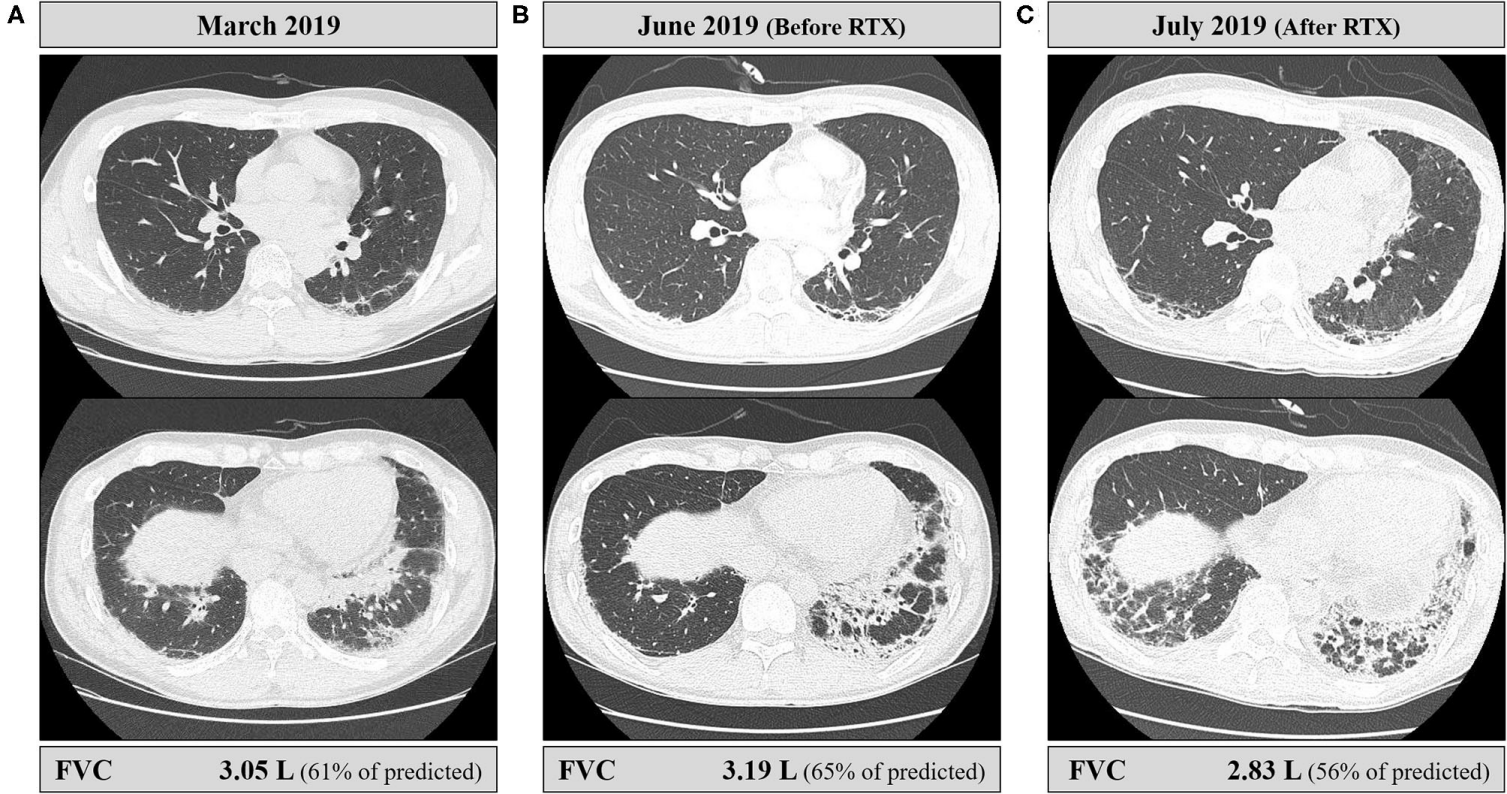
Serial chest CT findings and pulmonary functions of the patient. (A) Initial CT at the time of diagnosis of ILD showed perilobular consolidations and fibrosis in posterior aspects of the lower lobes, and forced vital capacity (FVC) was 3.05 L. (B) CT performed just before the rituximab injection showed the increased extent of fibrosis. However, the patient did not complain of respiratory symptoms including cough or dyspnea, and FVC was slightly improved by 4.6% compared to baseline. (C) CT performed immediately after the second rituximab injection showed an increased extent of fibrosis and perilobular consolidations. The patient developed mild dyspnea, and FVC declined by 11% from the latest one.
